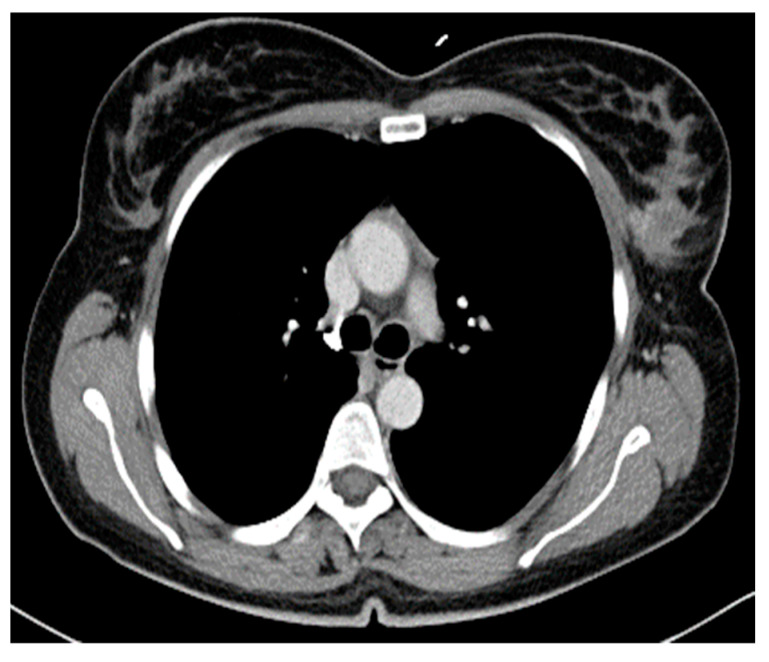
Left breast tumor CT aspect (institutional patient data).
